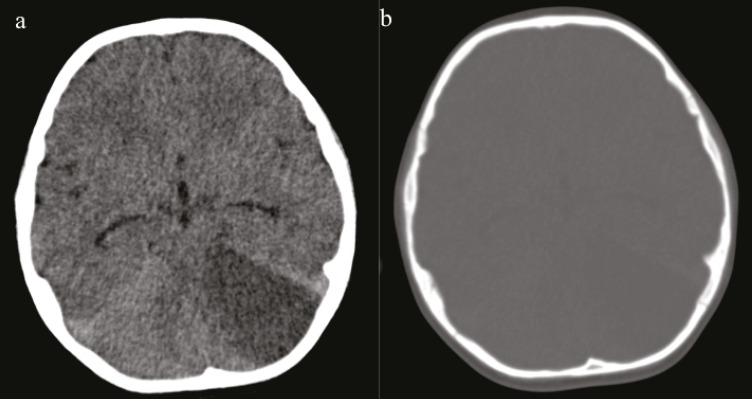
Non-contrast CT shows hypodens area with mass effect due to edema in the left cerebellar hemisphere (a). At the same level, there is no fracture of bone structure (b).
